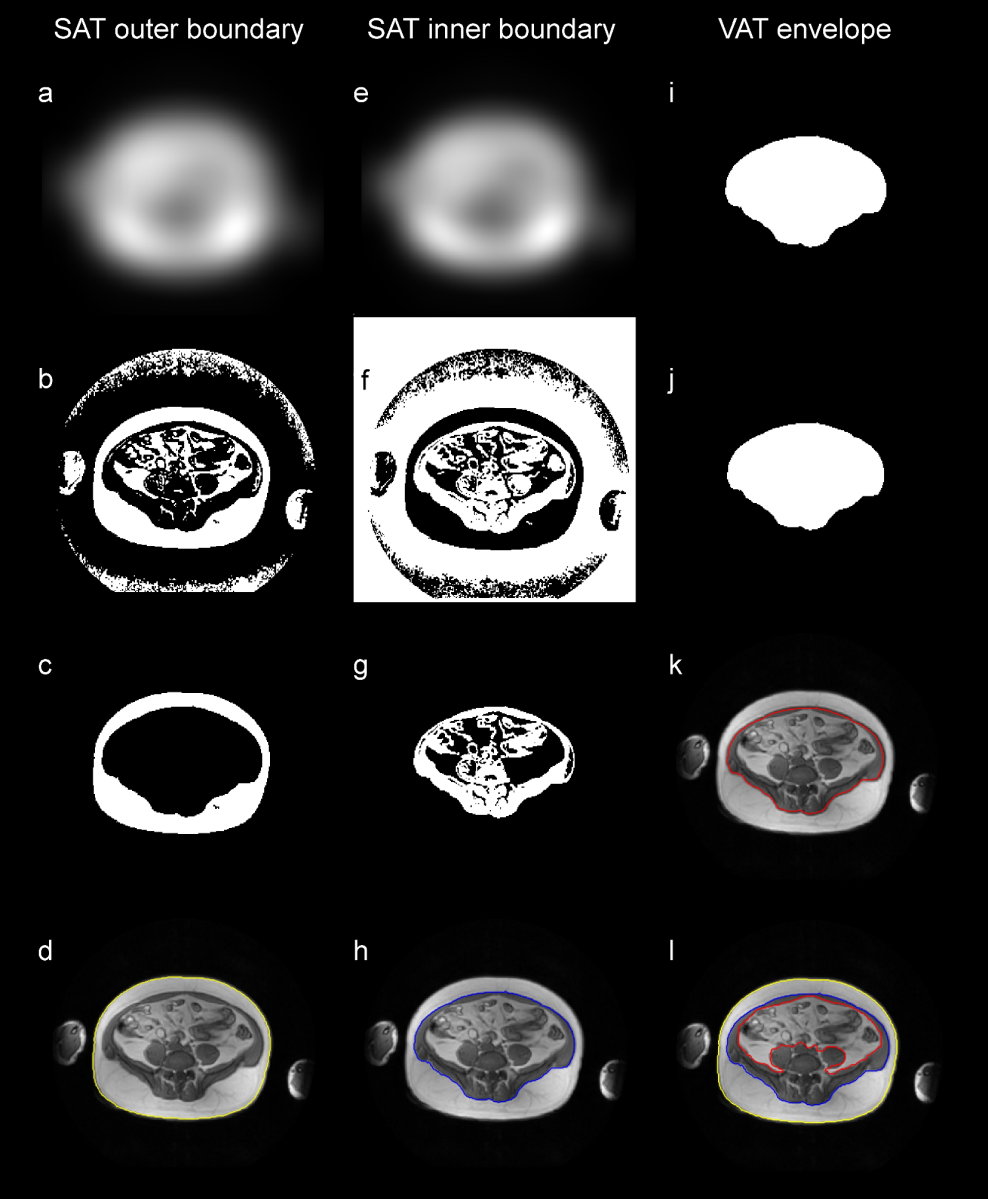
Main processing steps for computation of refined outer SAT (a-d, left column), refined inner SAT (e-h, middle column) and crude (envelope) VAT boundaries (i-l, right column). (a) adaptive threshold mask of MR image using *adaptthresh* (Matlab function) with filter size 19 × 19. (b) binarized MR image using *imbinarize* and mask from a. Resulting white areas primarily reflect adipose tissue but artefacts are present as well, most notably, at the bottom of the image. (c) largest connected white-pixel region from b determined using *regionprops* is considered as SAT. Further criteria were implemented for consistency, for example, the ratio of the principal diameters of considered regions. (d) resulting outer (yellow) SAT contour computed using *bwboundaries* on filled mask (not shown here) and overlaid on original MR image. (e) adaptive threshold mask with 17 × 17 filter used for binarization and (f) resulting binary mask (inverted). (g) largest connected region of mask f after multiplication with filled mask for outer SAT contour. (h) resulting inner (blue) SAT contour. (i) filled mask for inner SAT contour after evaluation of different threshold masks and quantitative criteria (region size and eccentricity). (j) eroded mask from i and (k) resulting envelope (red) boundary for VAT. (l) MR image annotated with all boundaries.
